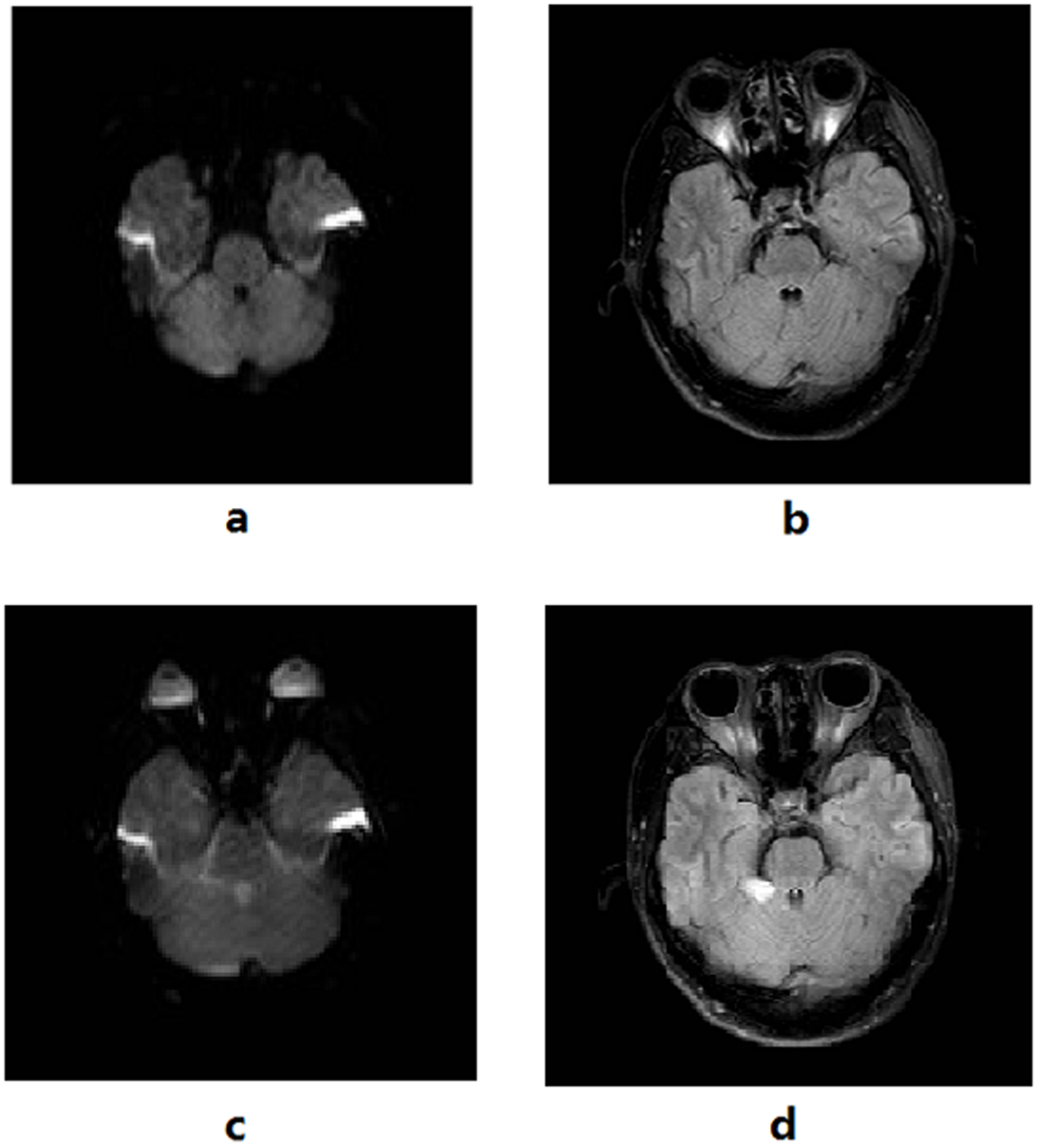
A 25-year old man with acute cerebral infarction received MRI within 2.5 hours of onset. His NIHSS score was 3 and MRI failed to reveal any positive findings. The right superior cerebellar artery was not visualized on cerebral MRA. The patient was stared with thrombolytic therapy and MRI after one week showed patchy ischemic infarct in the right cerebellum. Initial cerebral MRI (a) and FLAIR (b) at 2.5 hours after onset; repeat DWI (c) and FLAIR (d) at one week.
